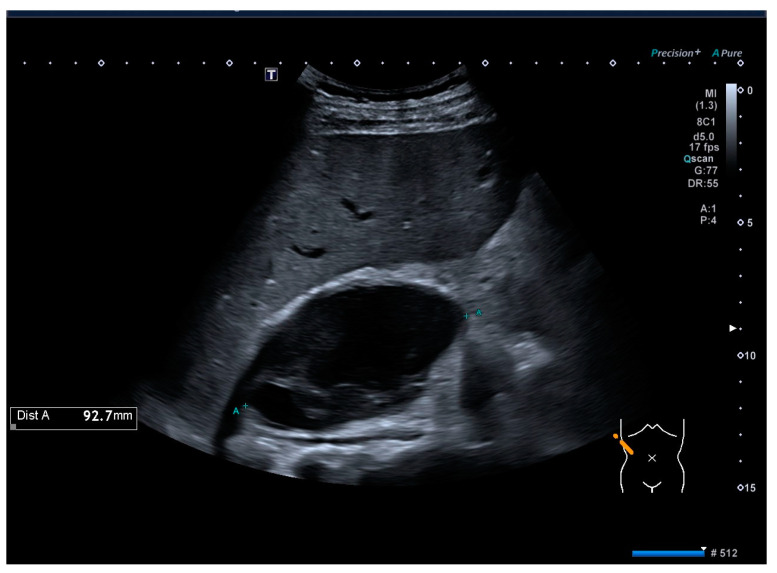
Abdominal ultrasound. A hematoma is visible in the projection of the right adrenal gland.
